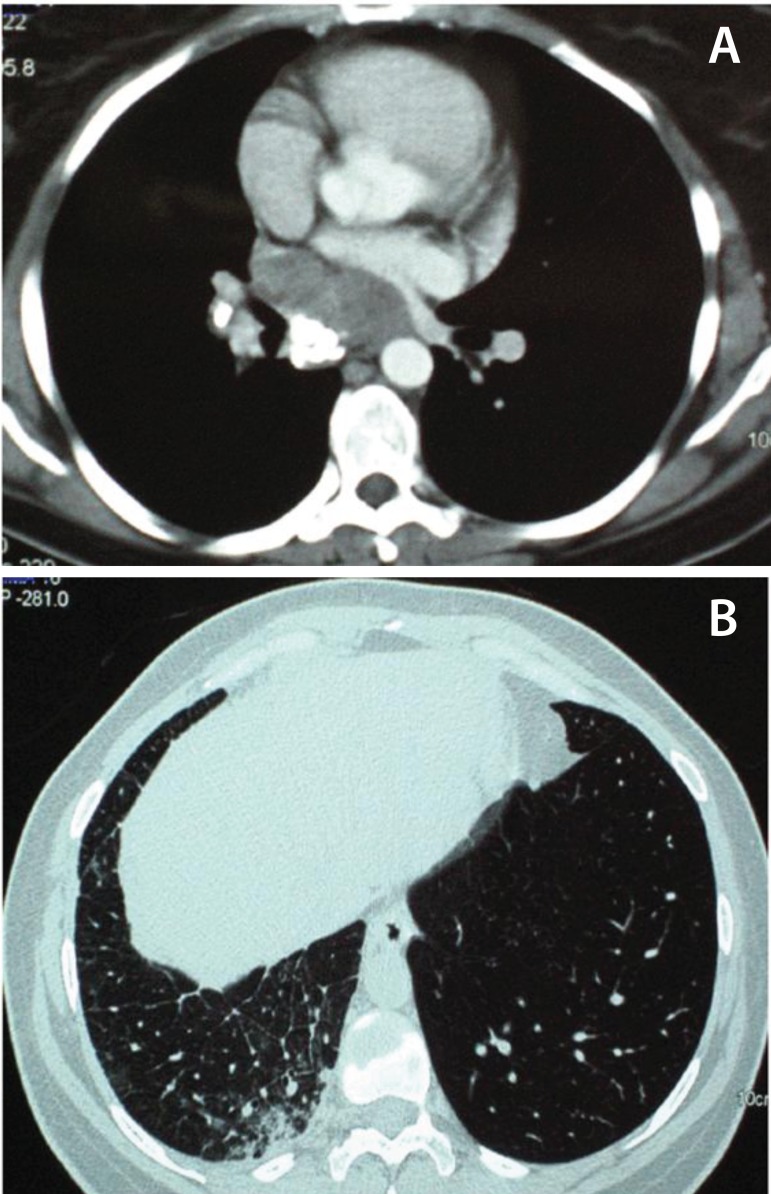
A: Axial contrast-enhanced lung CT (mediastinal windows): There is a soft
